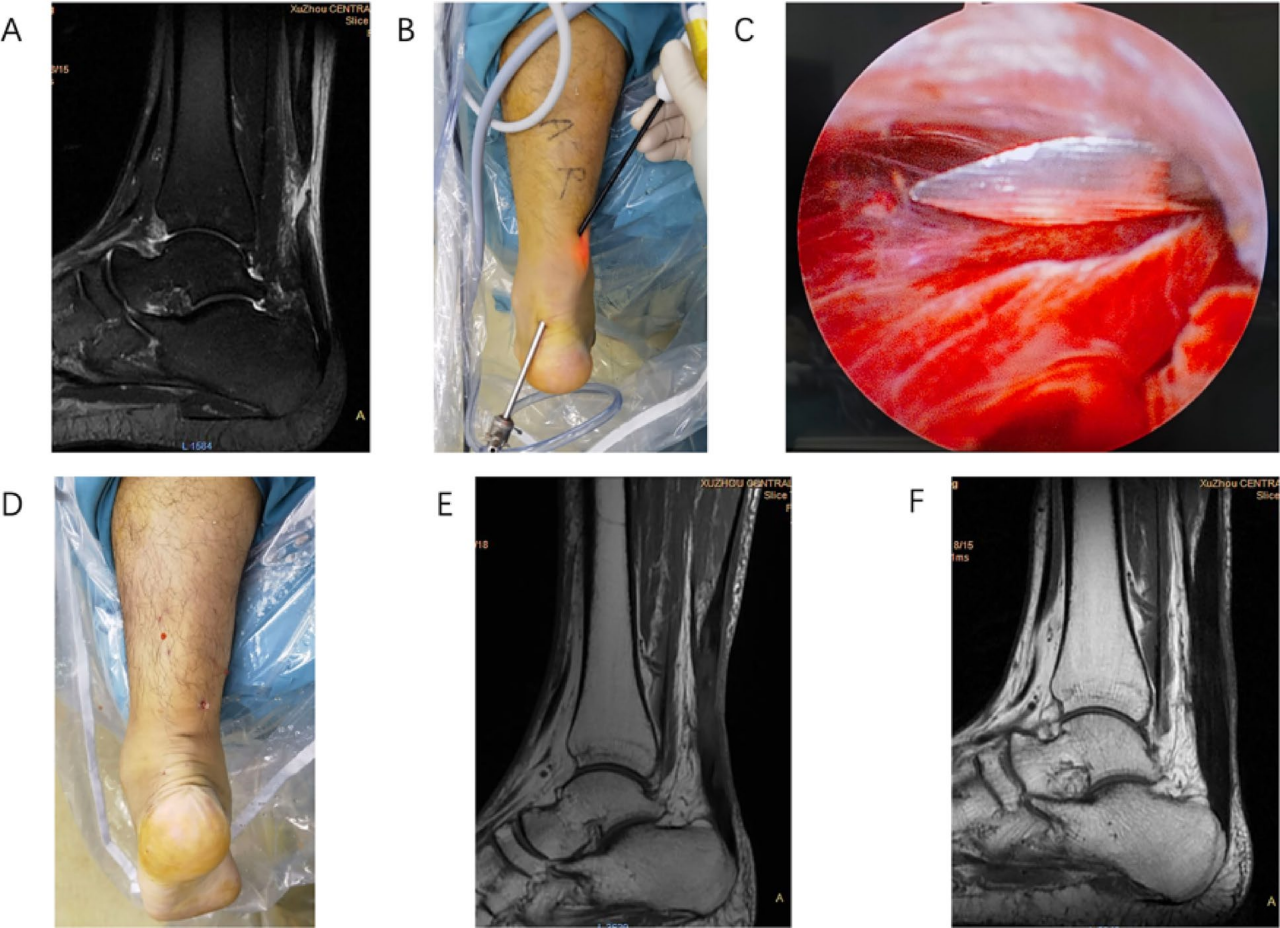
The two-portal all-inside endoscopic modified Bunnell suture configuration to repair an acute midsubstance Achilles tendon rupture on the left side. A acute midsubstance Achilles tendon rupture confirmed at magnetic resonance imaging (MRI); B overview of the endoscopic portals; C endoscopic view of the ruptured Achilles tendon with the suture needle passing through the paratenon; D appearance after repair; E MRI performed two months postoperatively: satisfactory tendon healing; F MRI obtained at six months postoperatively: well-integrated tendon healing
